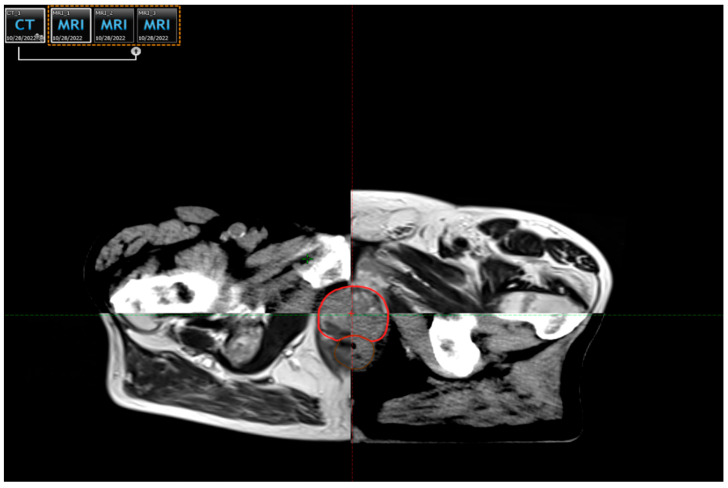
Radical prostate cancer radiotherapy using the VMAT technique: the clinical target volume (CTV), marked in red, was delineated using co-registered magnetic resonance imaging (MRI), ensuring precise mapping and accurate treatment delivery (material from the Institute for Oncology and Radiology of Serbia).
